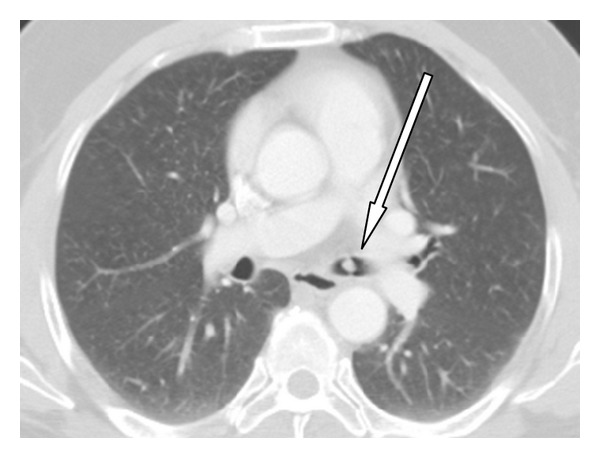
Axial CT of chest (lung window) at the level of left main pulmonary artery showing a pedunculated mass 0.5 cm in the left main bronchus (white arrow).
